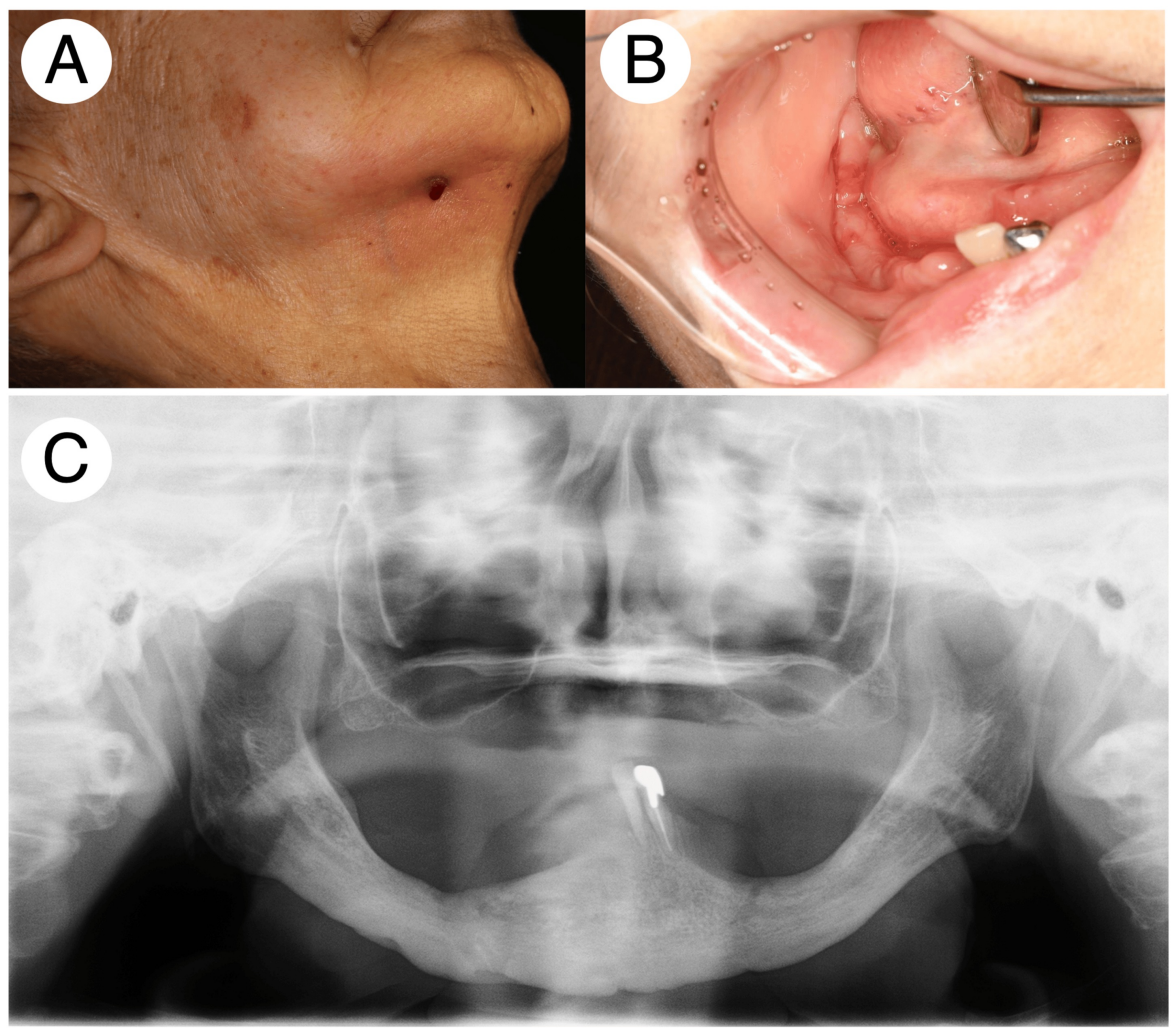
Patient 3 clinical and radiographic findings after the treatment. Extra- and intraoral examination detected the fistula covered with granulation tissue (A and B). Panoramic X-ray identified a mild increase in sclerotic changes in the mandibular bone without any signs of periosteal reaction (C).
